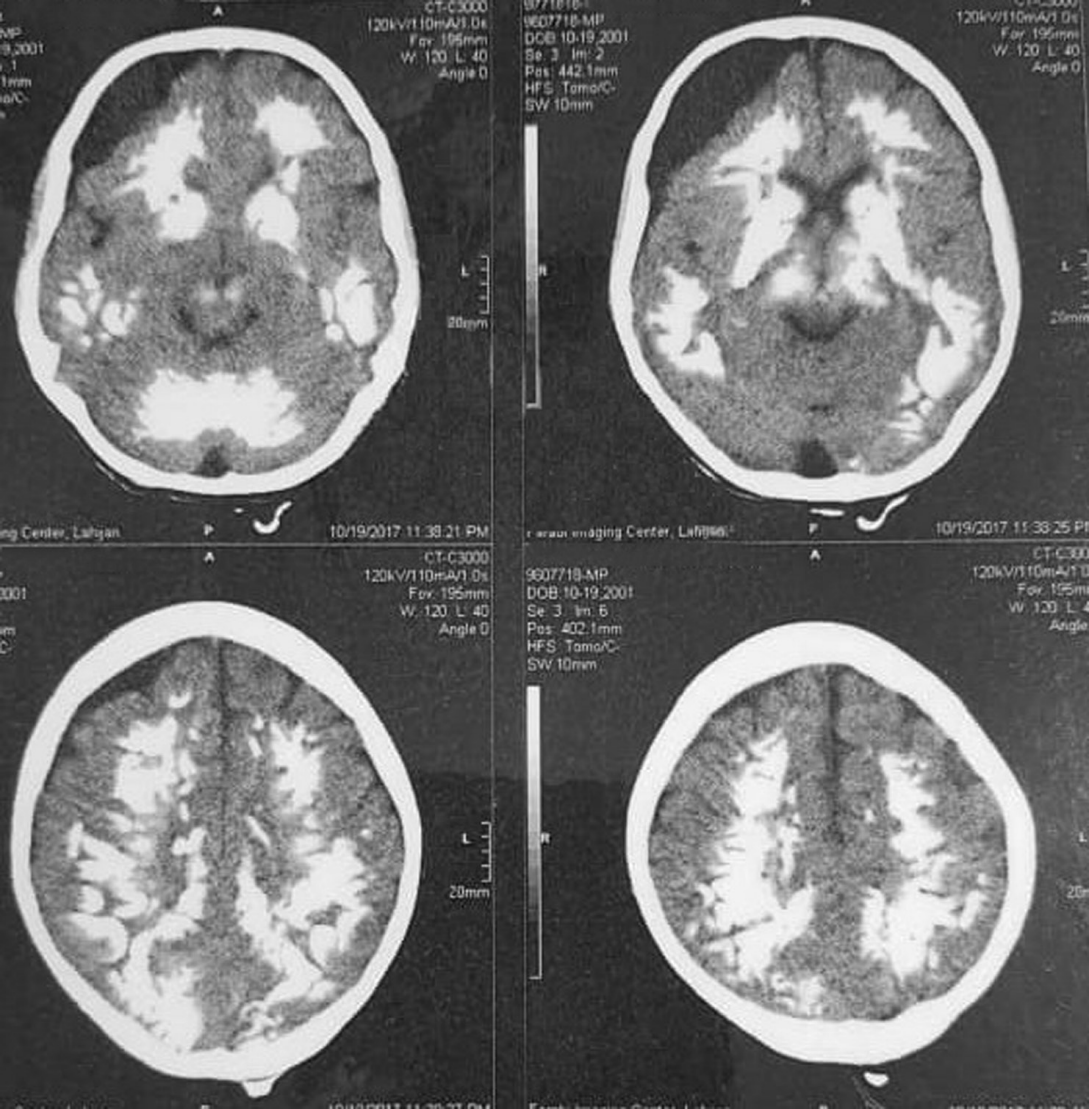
Axial planes of brain CT scan of the proband case demonstrate diffuse symmetrical calcifications together with subdural effusion in right side
